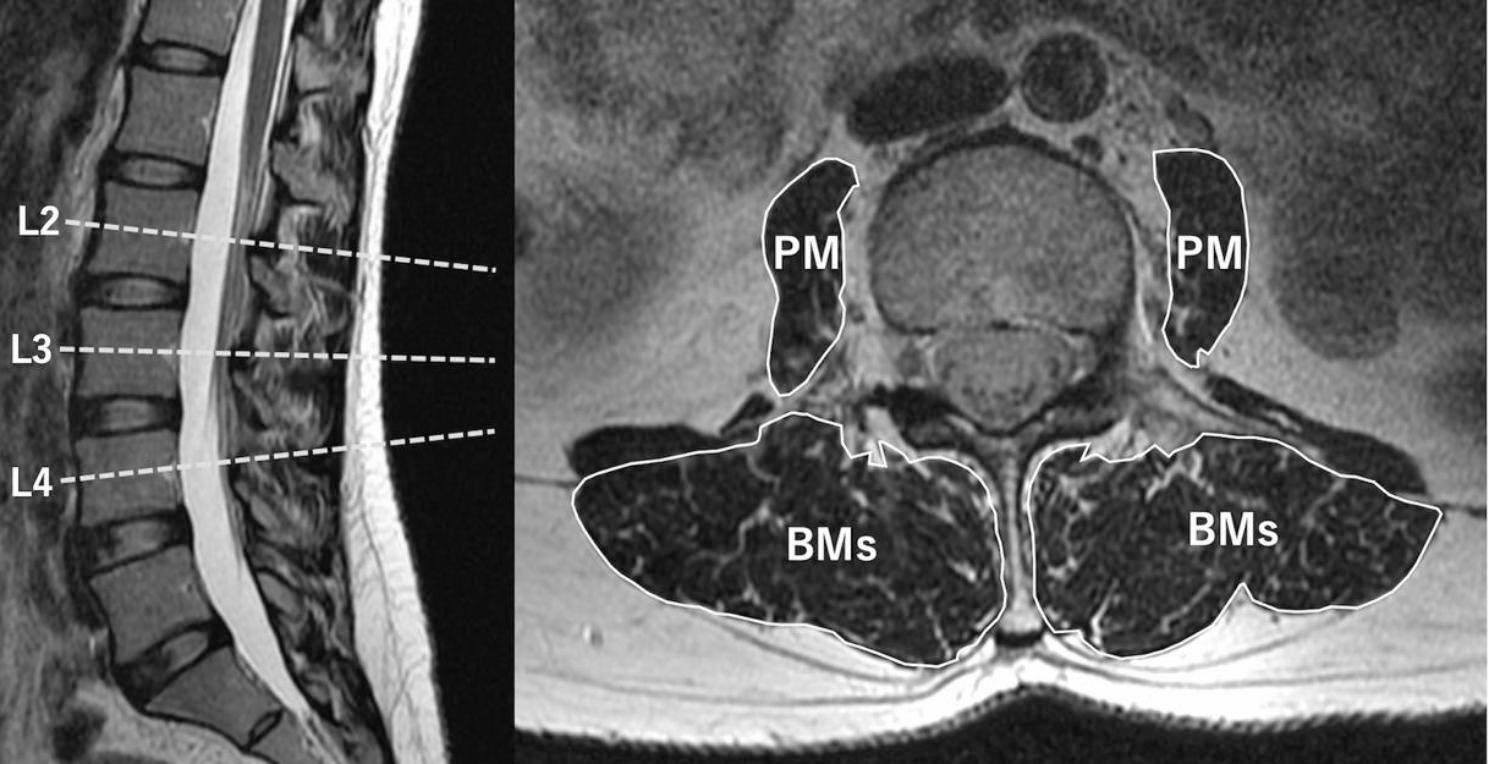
Cross-sectional areas (CSA) of the psoas major (PM) and back muscles (BMs) CSA of the PM and BMs were obtained bilaterally in the middle of L2, L3, and L4 on T2-weighted lumbar axial MR images
